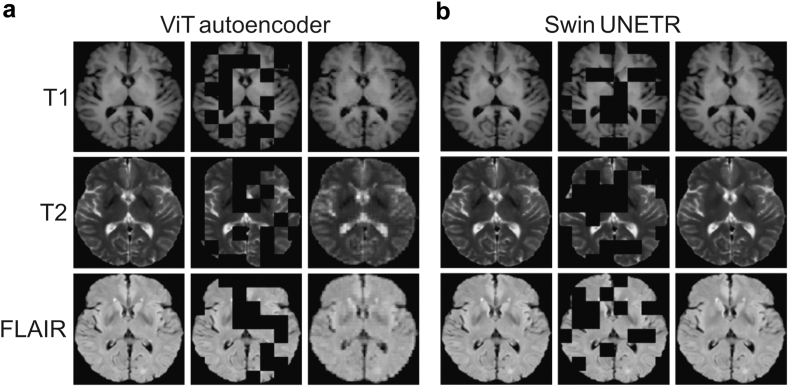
Reconstruction of a representative case from the pre-training dataset by the ViT autoencoder (a) and Swin UNETR (b). Abbreviations: T1, T1-weighted imaging; T2, T2-weighted imaging; FLAIR, fluid attenuated inversion recovery; Orig, origin; Recon, reconstruction.
